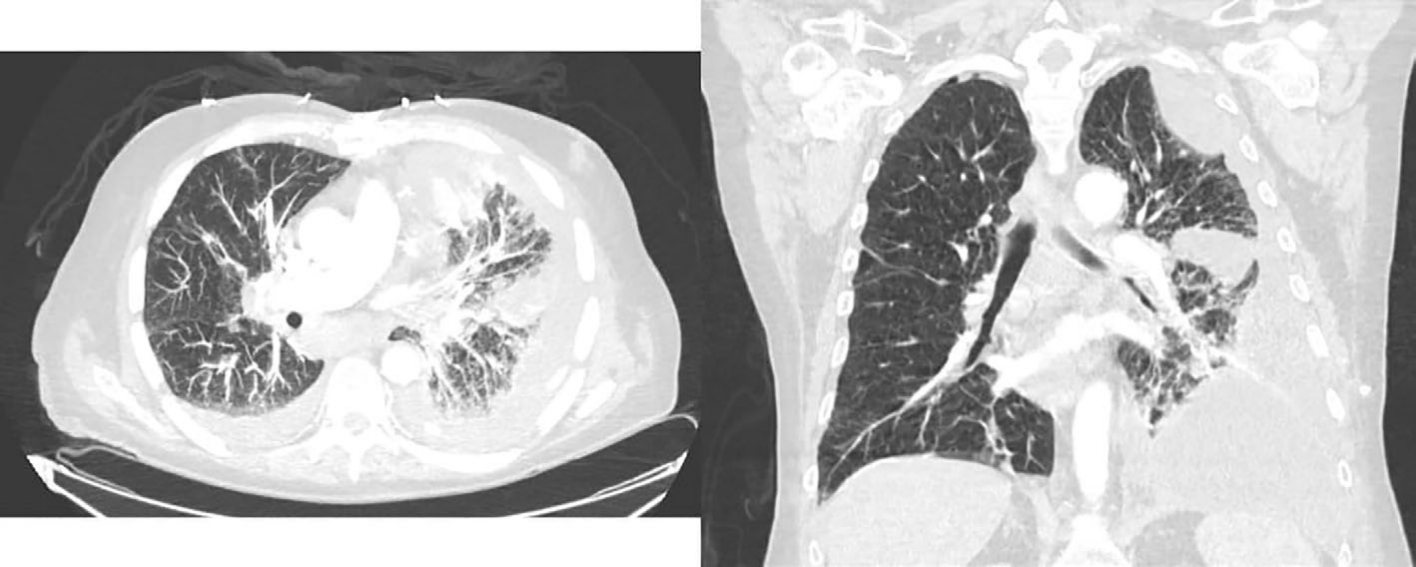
Transverse and coronal view of chest CT showing loculated left‐sided pleural effusion
